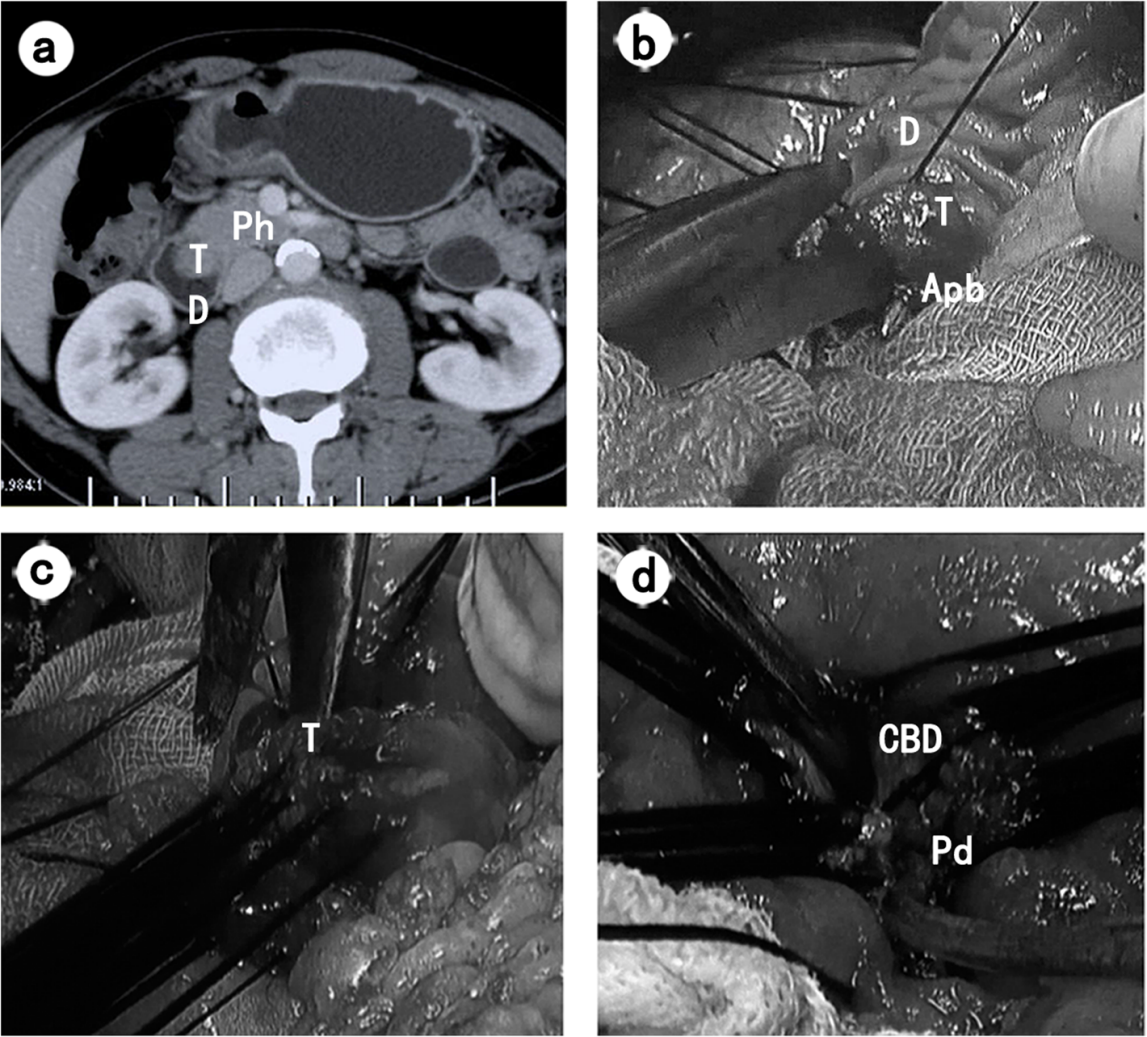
Main surgical technique of TDA. a Preoperative CT image showed the ampullary tumor protruded into duodenal lumen as a bulging. b Intraoperative photograph showed that local resection of the tumor started from the site of the 11 o’clock position. c Intraoperative photograph showed the circumferential resection of the tumor was about 5–10 mm from the edge of the tumor. d Intraoperative photograph showed the reconstructed ampullary region. *T* ampullary tumor; *D* duodenum; *Ph* pancreatic head; *Apb* ampullo-pancreatobiliary common duct; *CBD* common biliary duct; *Pd* pancreatic duct
